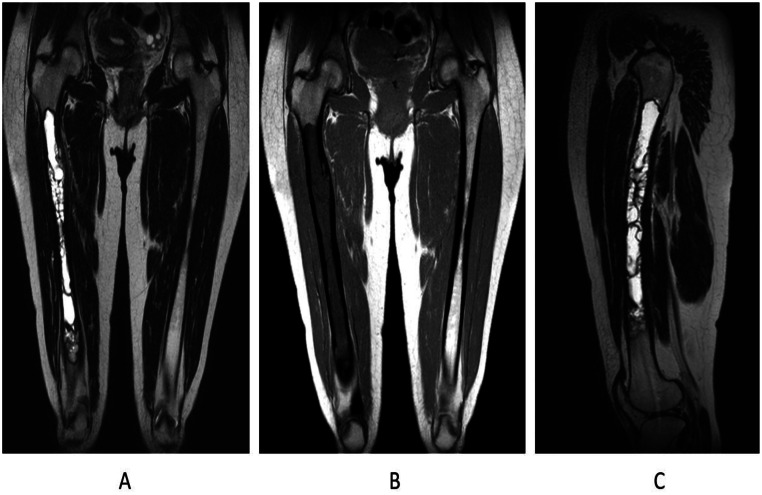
MRI of the right lower limb in coronal section T2 (A), coronal T1 (B) and sagittal section T2 (C): Centromedullary lesional process in the right femoral diaphyseal (25 cm of height) composed of many cystic formations, in low signal T1 and high signal T2, not enhanced after contrast.
